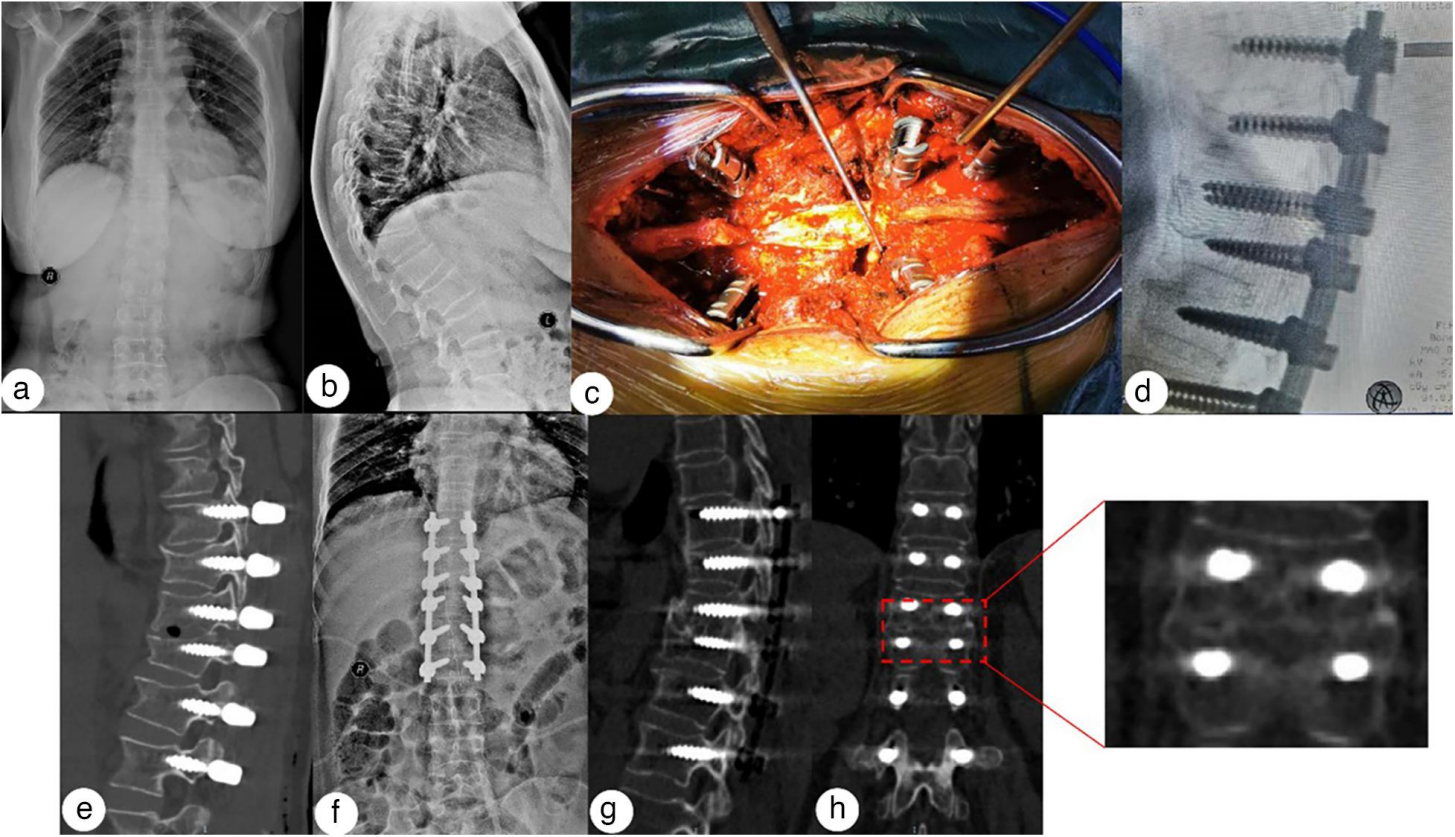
A 51-year-old woman who developed kyphosis after an L1 fracture caused by a fall. The VAS score was 8. (a–c) A preoperative X-ray shows a long-standing compression fracture of the L1 vertebral body, with a kyphosis Cobb angle of 61.93°. (d–f) The postoperative correction was satisfactory, with a kyphosis Cobb angle of 11.50°. (g,h) At the 2-year follow-up, there were no complications such as nail or rod breakage. The osteotomy surface was fused, and the kyphosis Cobb angle was 23.04°.
